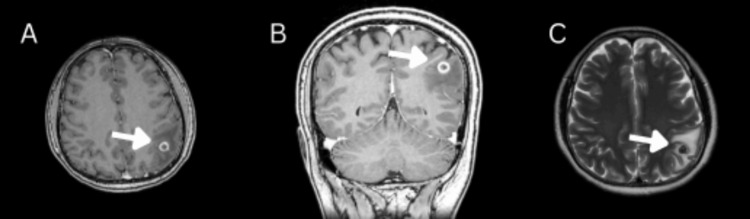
MRI brain showing a ring-enhancing lesion in the left parietal lobe with surrounding edema, as indicated by the white arrow. (A) Axial T1-weighted post-contrast image, (B) coronal T1-weighted post-contrast image, and (C) axial T2-weighted image. The lesion demonstrates a hypointense center with an enhancing rim on T1 post-contrast sequences and surrounding hyperintense edema on T2-weighted imaging, consistent with a calcified granuloma.
